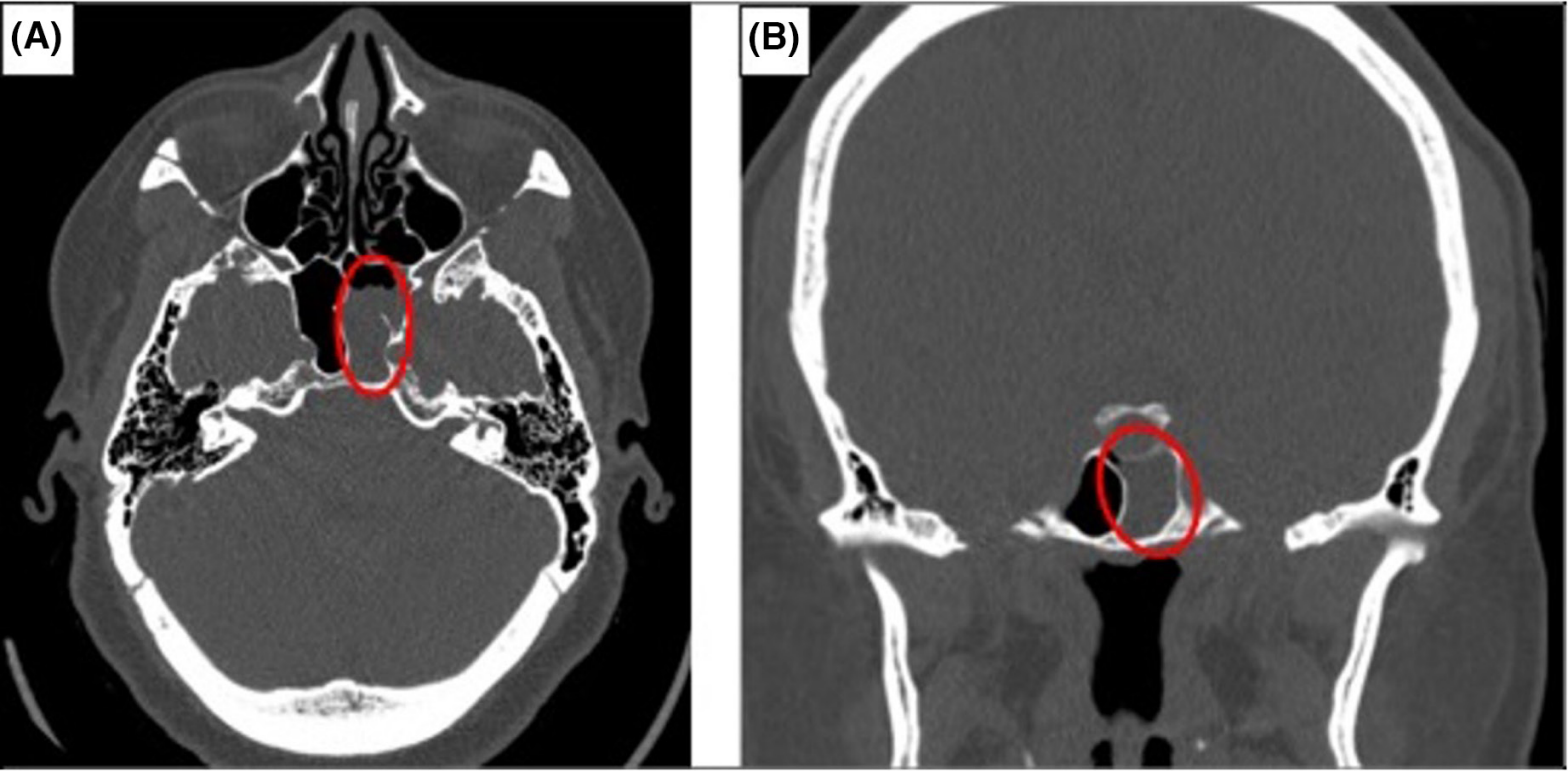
(A) Axial and (B) Coronal views of CT scan depict prominent air fluid level in the left sphenoid sinus extension (circled).
